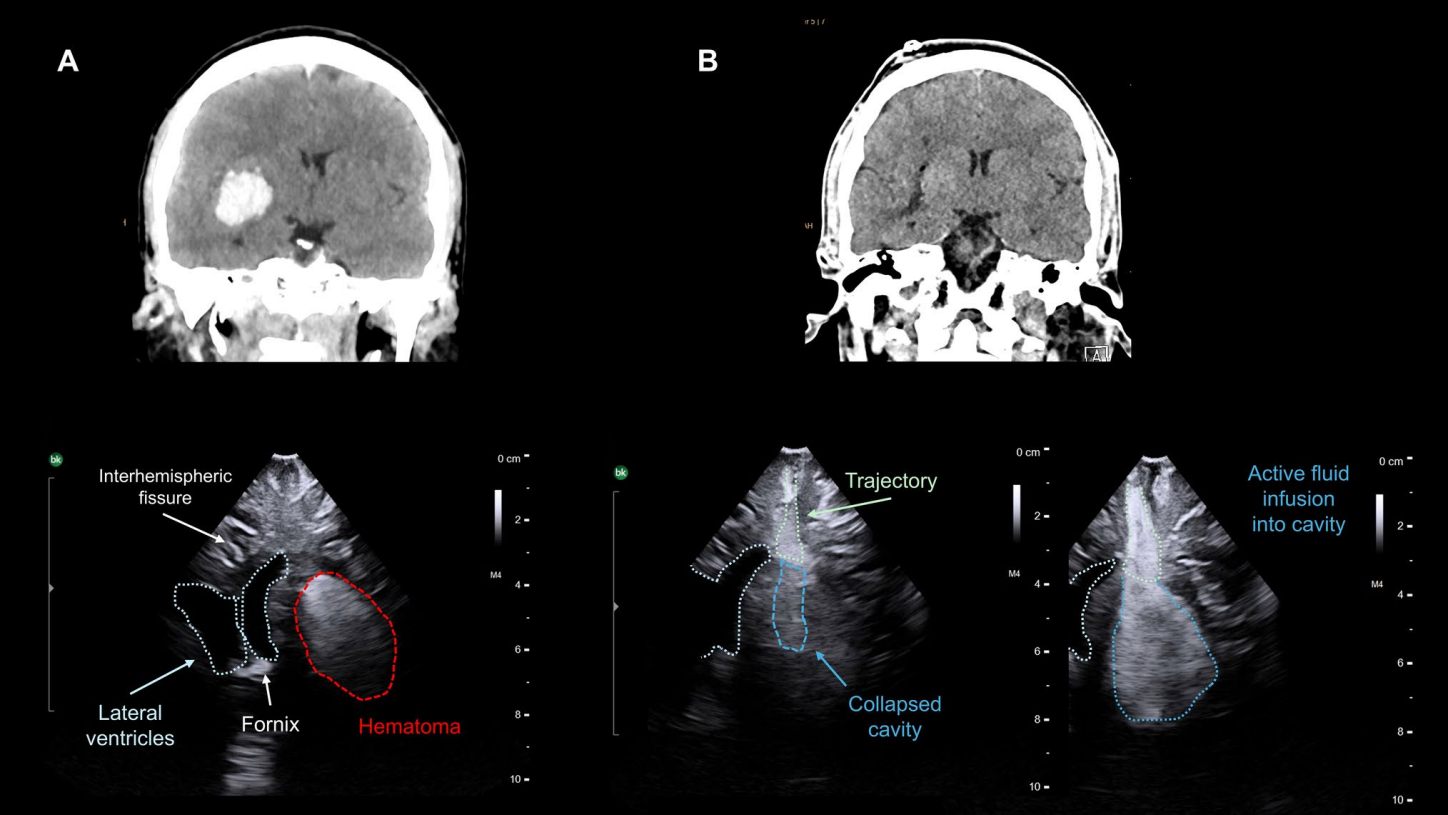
Complete evacuation of the hematoma as seen with intraoperative US. A Above, the basal CT scan showing a 20 cm3 right basal ganglia hematoma. Below, the corresponding US image obtained through a mini-craniotomy before opening the dura. Note that the lateral ventricles can be used for orientation in order to identify the hematoma. The hematoma appears as an hyperechogenic oval structure; the deeper portion may be hypoechogenic due to an acoustic shadow effect. B Above, postoperative CT showing complete hematoma evacuation after one pass of aspiration. Below, images obtained with US after the first pass of aspiration. On the left, the cavity is collapsed, and the acoustic signal is similar to the surrounding parenchyma. On the right, the cavity is being filled with saline solution infused with a syringe through the trajectory path. Note the hyperechogenic and homogeneous appearance of the dilated saline-filled cavity
